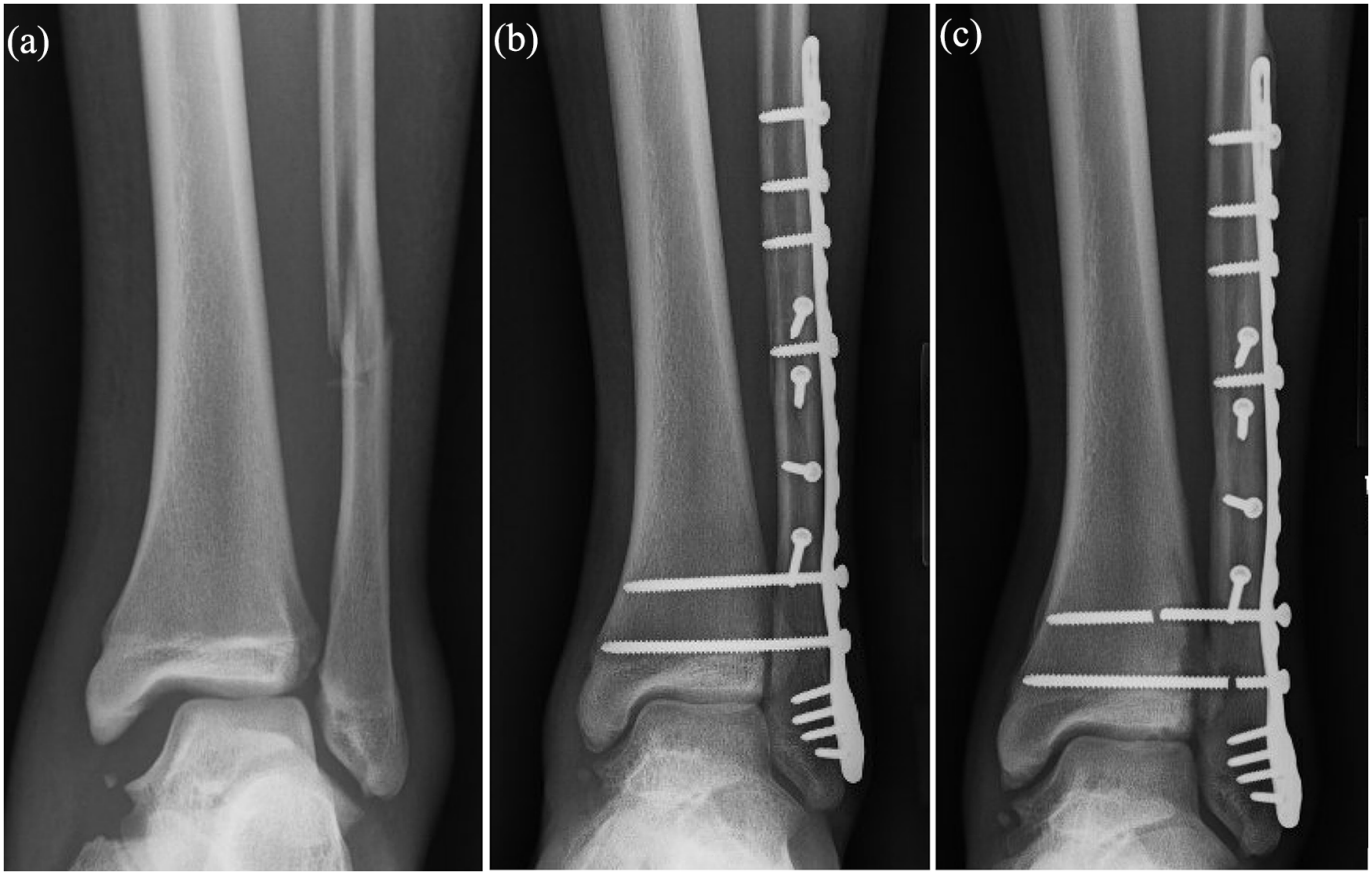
(a) Radiograph of Weber C type distal fibula fracture with medial clear space and tibiofibular clear space widening, (b) immediate postoperative radiograph of fibular plate and screw fixation construct and double syndesmotic screw fixation, and (c) 8-month postoperative radiograph demonstrating syndesmotic screw breakage requiring revision surgery.
